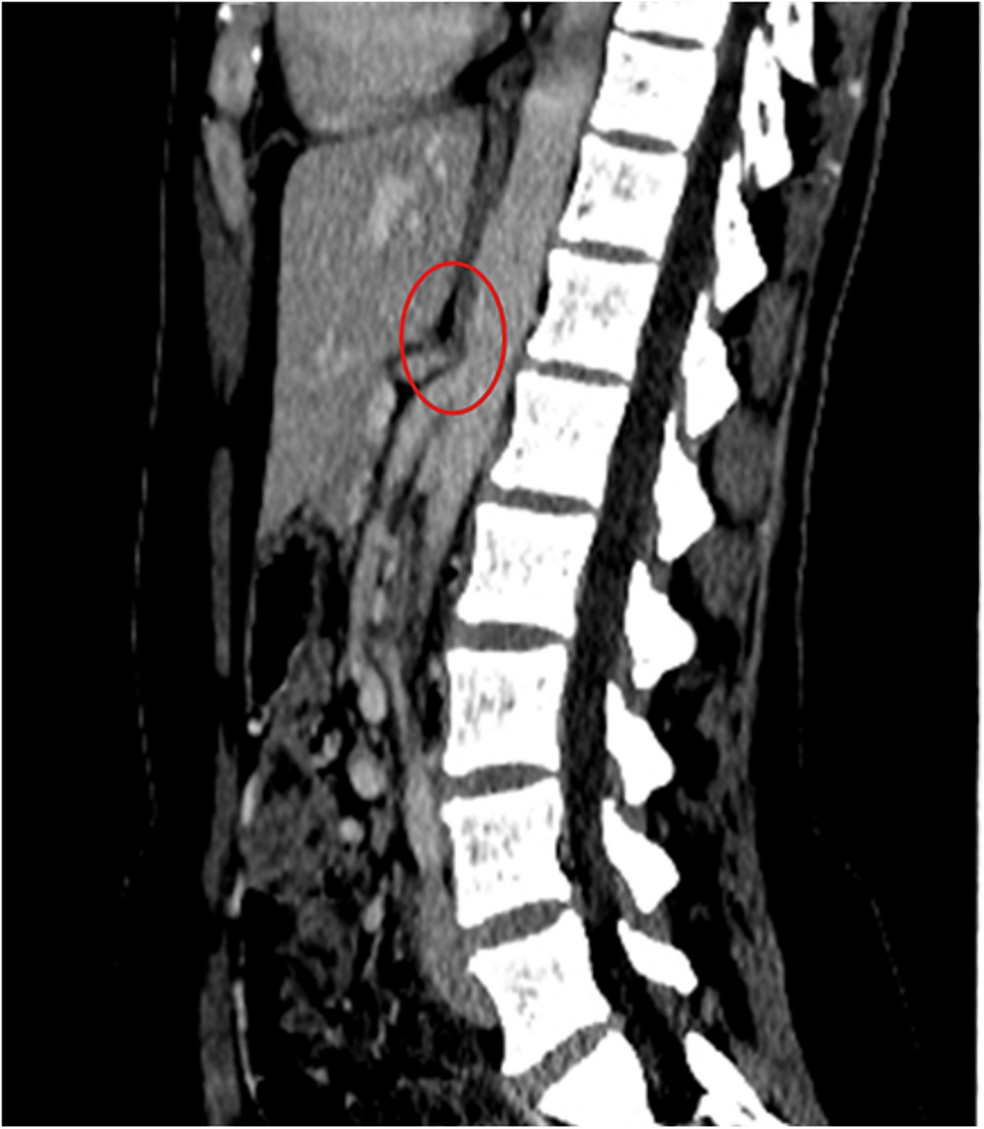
CT (sagittal plane) showing a hook-like sign CT: computed tomography
